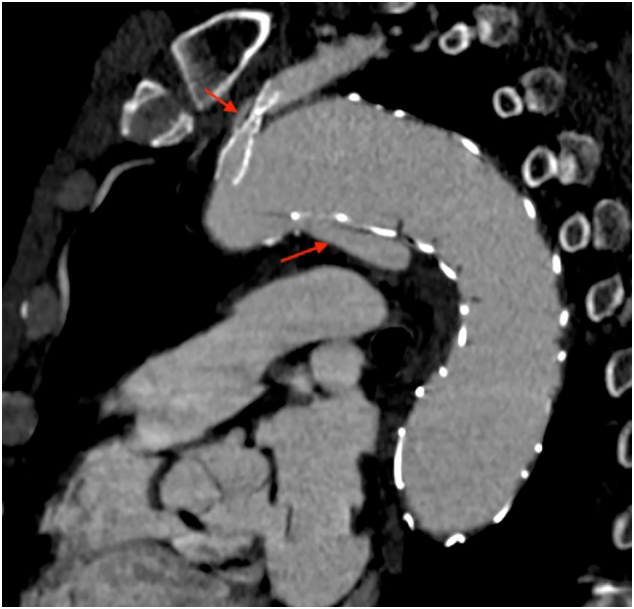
Follow-up 15 months CT-angiography showing deterioration of penetrating aortic ulcer and crashing of stent in left subclavian artery.
